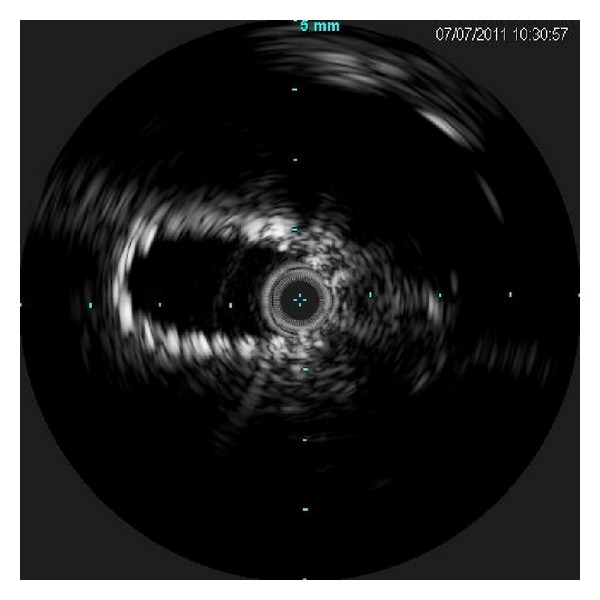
Poststent placement intravascular ultrasound (IVUS) in the left iliac improvement in lumen size.
